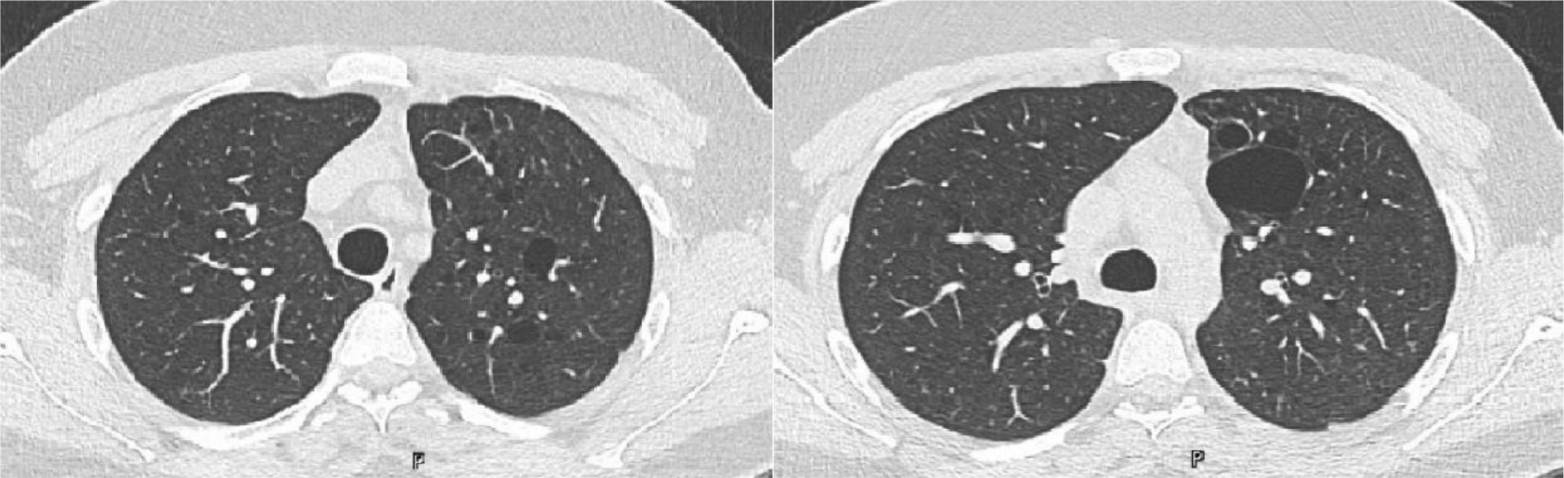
CT chest image showing bilateral cystic lung changes, with the left lung showing more prominent cysts.
